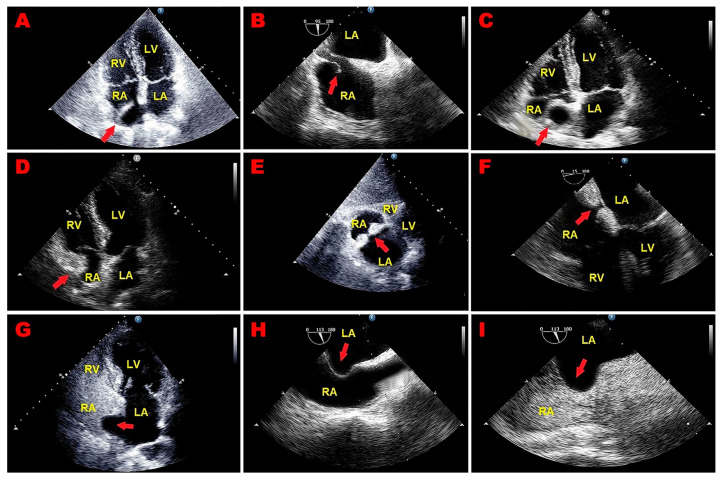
Representative examples of echocardiographically detected RA pseudomasses. (A) Transthoracic echocardiography. Apical four-chamber view, showing prominent Eustachian valve (red arrow). (B) Transesophageal echocardiography. Mid-esophageal bicaval view, revealing prominent Eustachian valve (red arrow). (C) Transthoracic echocardiography. Apical four-chamber view, revealing redundant Chiari’s network (red arrow). (D) Transthoracic echocardiography. Apical four-chamber view, highlighting lipomatous atrial hypertrophy involving RA free wall (red arrow). (E) Transthoracic echocardiography. Subcostal four-chamber view, demonstrating lipomatous atrial septal hypertrophy (red arrow). (F) Transesophageal echocardiography. Mid-esophageal four-chamber view, showing lipomatous atrial septal hypertrophy (red arrow). (G) Transthoracic contrast echocardiography. Apical four-chamber view, showing an atrial septal aneurysm (red arrow) protruding into the RA cavity, mimicking an RA mass, with no evidence of interatrial shunt on saline contrast echocardiography. (H) Transesophageal echocardiography. Mid-esophageal bicaval view, showing an atrial septal aneurysm (red arrow) protruding into the RA cavity, mimicking an RA mass. (I) Transesophageal contrast echocardiography. Mid-esophageal bicaval view, highlighting atrial septal aneurysm (red arrow) protruding into the RA cavity with no evidence of interatrial shunt on saline contrast echocardiography. LA, left atrium; LV, left ventricle; RA, right atrium; RV, right ventricle. (A–C,E–I) are reproduced from the paper [79].
